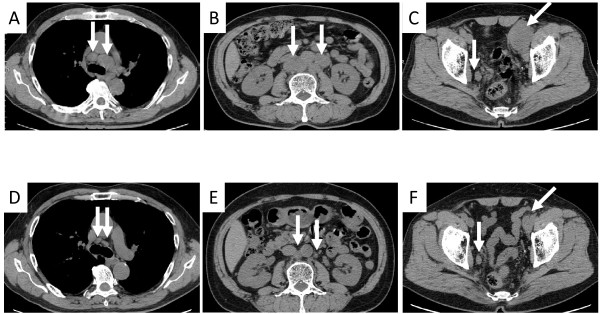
Computed tomography before and after treatment. Pre-treatment images showed enlarged mediastinal (A, arrows), para-aortic (B, arrows), and iliac (C, arrows) lymph nodes. Images nine months after ADT showed a partial response of the mediastinal (D, arrows), para-aortic (E, arrows), and iliac (F, arrows) lymph nodes.
